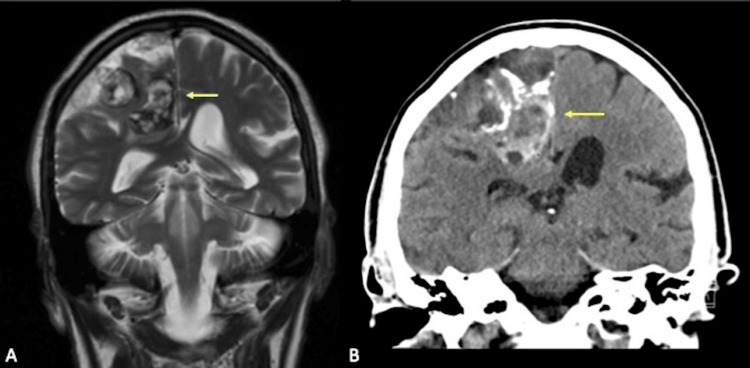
Coronal CT and MRI sequences A: Coronal T2-weighted sequence demonstrates a heterogeneous lesion in the right frontoparietal region with peripheral T2 hypointensity, correlating to calcification. B: Coronal CT shows hyperattenuation in the right frontoparietal lesion and calcification, correlating to T2 hypointensity on MRI.
